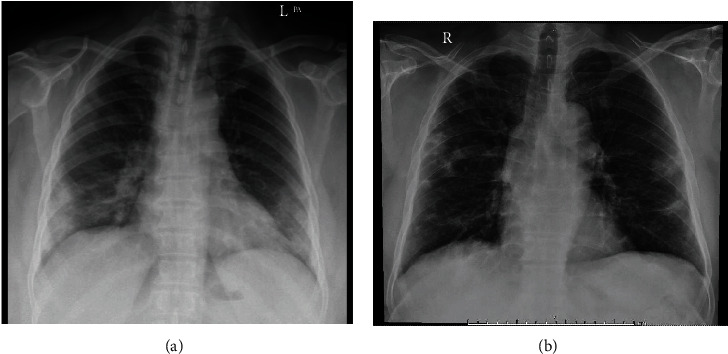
Chest x-rays of two patients (a and b). (a) Peripheral focal areas of ground glass opacities and consolidation at bilateral middle and lower lung zones. (b) Peripheral areas of ground glass opacities and consolidation at right upper, bilateral middle, and lower lung zones.
